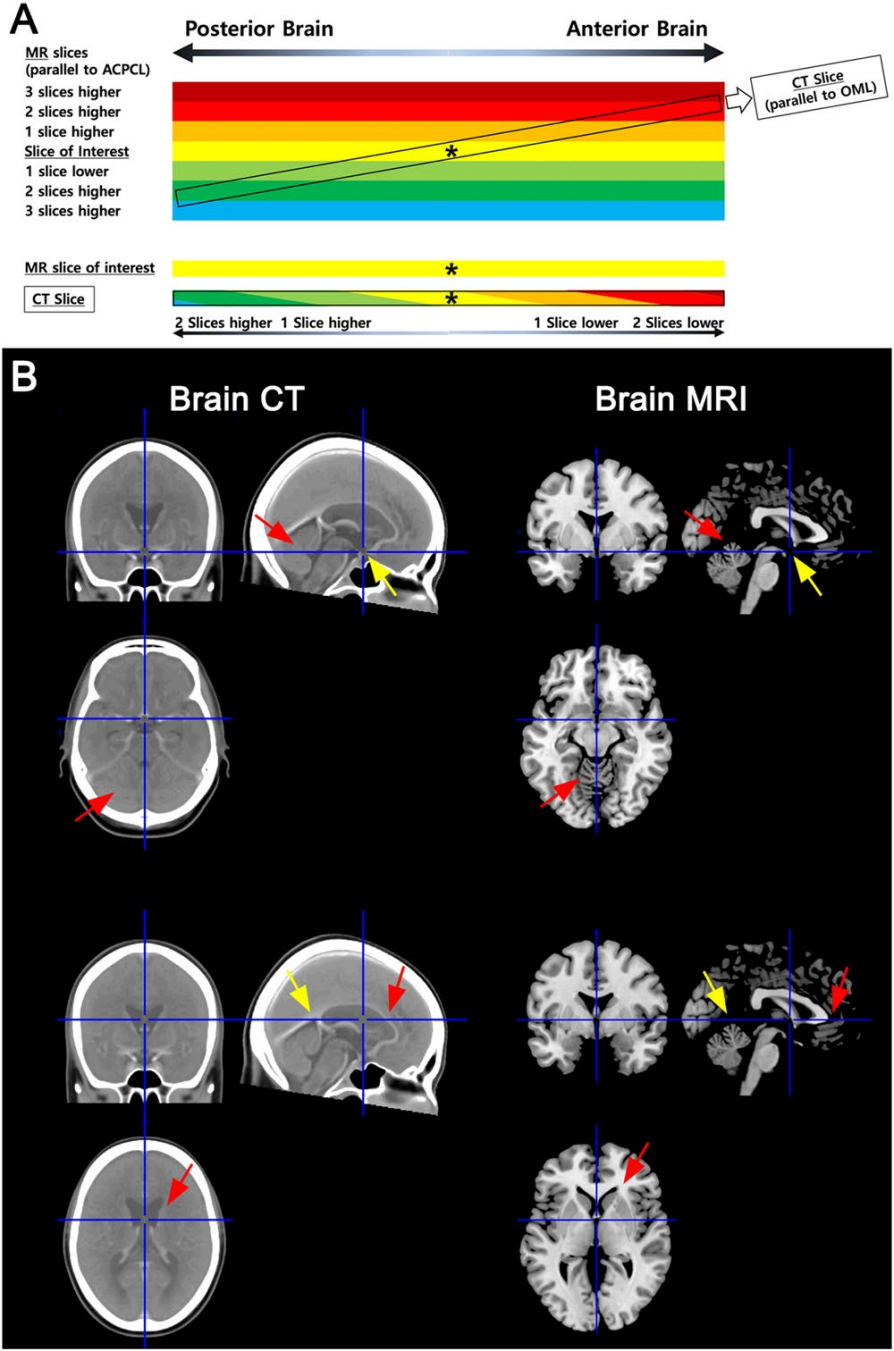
Different slice angles for axial MR vs. CT images. (A) The anterior-posterior commissure line (ACPCL) and orbitomeatal line (OML) for the image slicing of respectively MRI and CT are not parallel. Because of the pitch angle difference (average 9°) between the imaging reference lines, the anterior and posterior portions of the axial CT image is respectively about ~10 mm lower and higher in the z-axis, with the central portion overlapping around the rotation point, centered on the MR slice of interest (asterisks). Here, 10-mm difference corresponds to about 2-slice difference, because the slice thicknesses of MRI and CT are respectively 6 mm and 5 mm. (B) Anatomical matching of the anterior portions in the MR and CT images (yellow arrows in the upper panel figures) causes a mismatching of the posterior portions (red arrows in the upper panel figures), and vice versa (yellow and red arrows in the lower panel figures).
